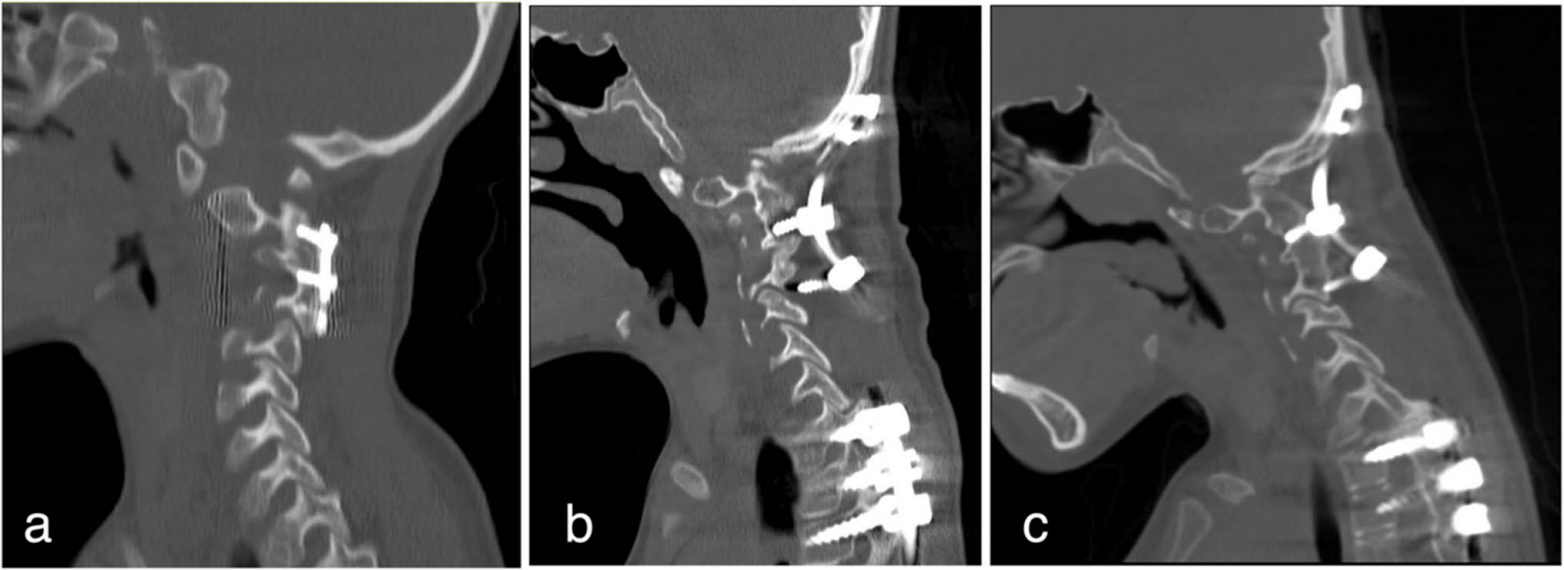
a Cervical spine CT at age 3 showing initial C2–C4 anterior–posterior fusion with anterior plating and posterior instrumentation, moderate kyphosis, and complete facet fusion. b Cervical spine CT at age 8 demonstrating occipital-cervical fusion with occipital plating and instrumentation to C4, screw loosening, and basilar invagination. c Cervical spine CT at age 9 revealing complete C2–C4 vertebral body fusion and C1–C5 posterior fusion with resected C2–C5 spinous processes
